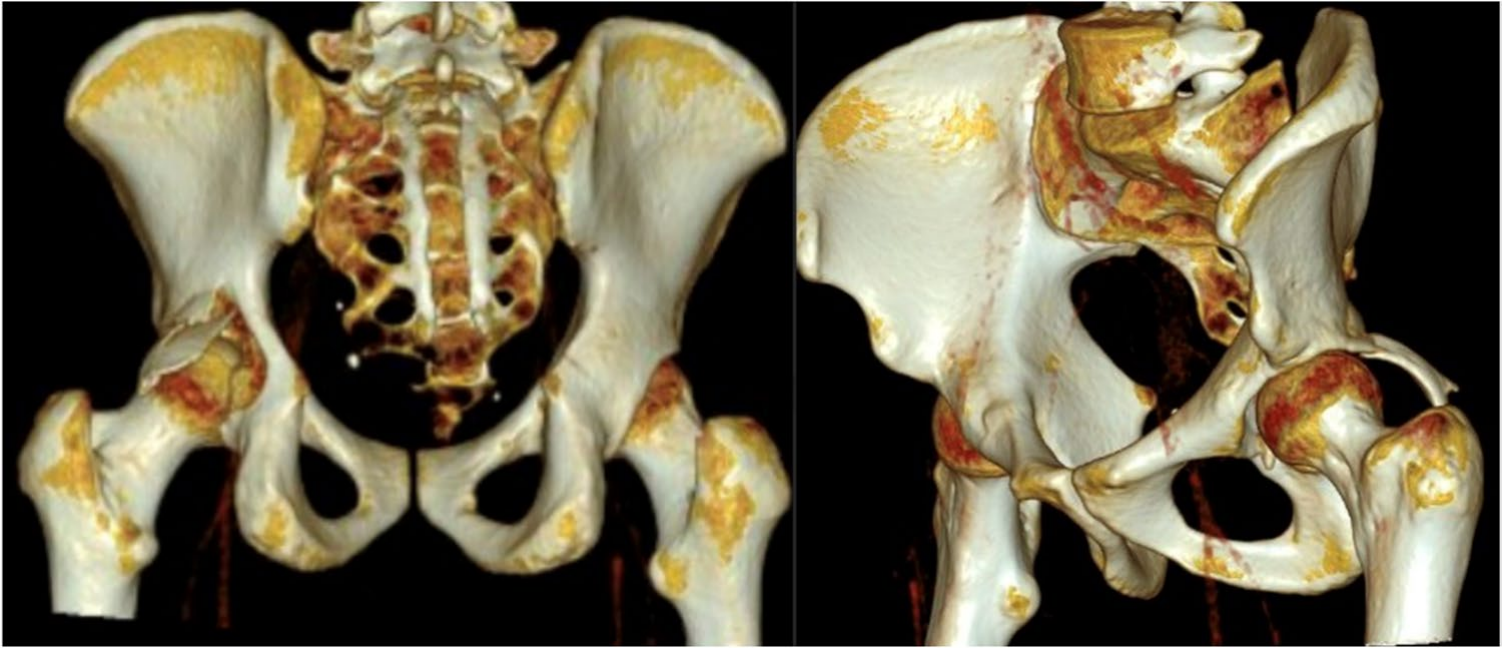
Three-dimensional CT scan for a patient with posterior wall fracture
